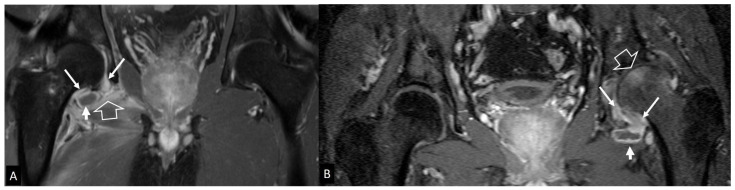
(A) Coronal fat-suppressed contrast-enhanced T1-w MR image in a 65-year-old male with staphylococcal septic arthritis, showing effusion (short arrow), synovitis (arrows), and soft-tissue edema (open arrow). (B) Coronal fat-suppressed contrast-enhanced T1-w MR image in a 59-year-old male with rheumatoid arthritis with left hip joint involvement, showing effusion (short arrow), synovitis (arrows), and bone marrow edema (open arrow).
